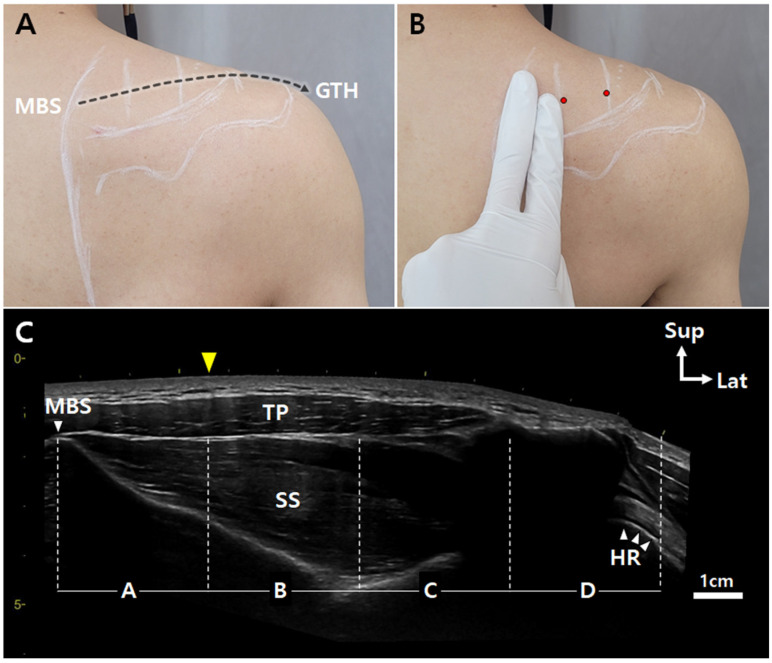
Injection guidelines based on surface landmarks. Ultrasonography scanning was performed from the MBS to GTH (A). Location of the injection sites using two fingers from the MBS (B). The panoramic view of ultrasonography image showing the four divisions of the SS. The location of 1/4 of the SS can be easily predicted with two fingers (C). HR: humerus; TP, trapezius; yellow arrowhead, right next to the second finger; dotted black arrow, ultrasonography scanning site; red circles, injection points; Sup, superior; Lat, lateral.
